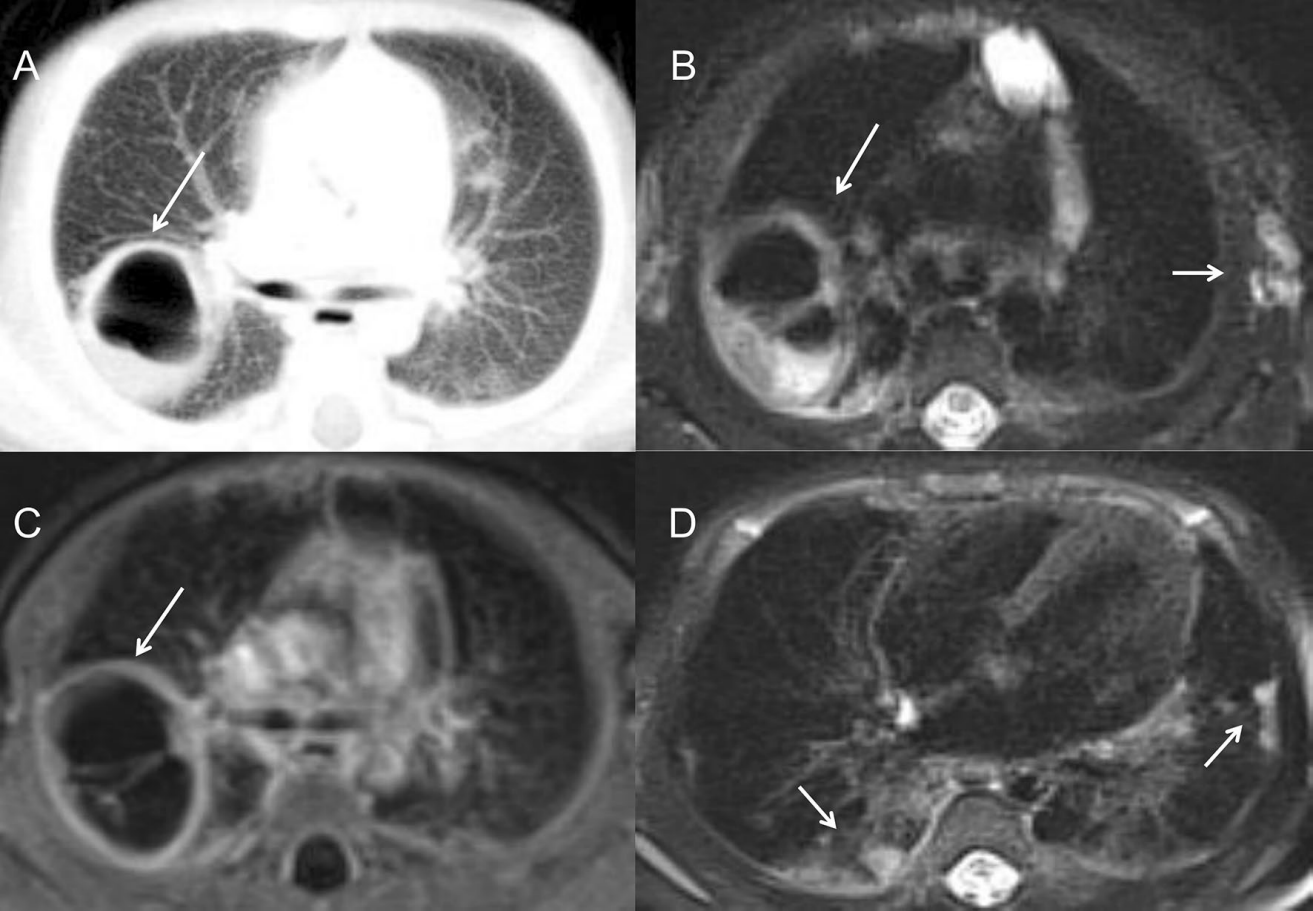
A 1-month-old boy with aspergillus infection. (A) On chest CT, a large cavity with thin and smooth wall was found in the right upper lobe, ranging 3 cm in diameter (long arrow). (B) On T2WI image, the linear separations and gas liquid leveling were found inside (long arrow). Multiple enlarged lymph nodes were found in the left axillary area (short arrow). (C) On post-contrast T1WI image, the wall of the cavity was obviously enhanced (long arrow). (D) Multiple pulmonary nodules were also seen in the bilateral lower lobe beneath the pleura (short arrow).
